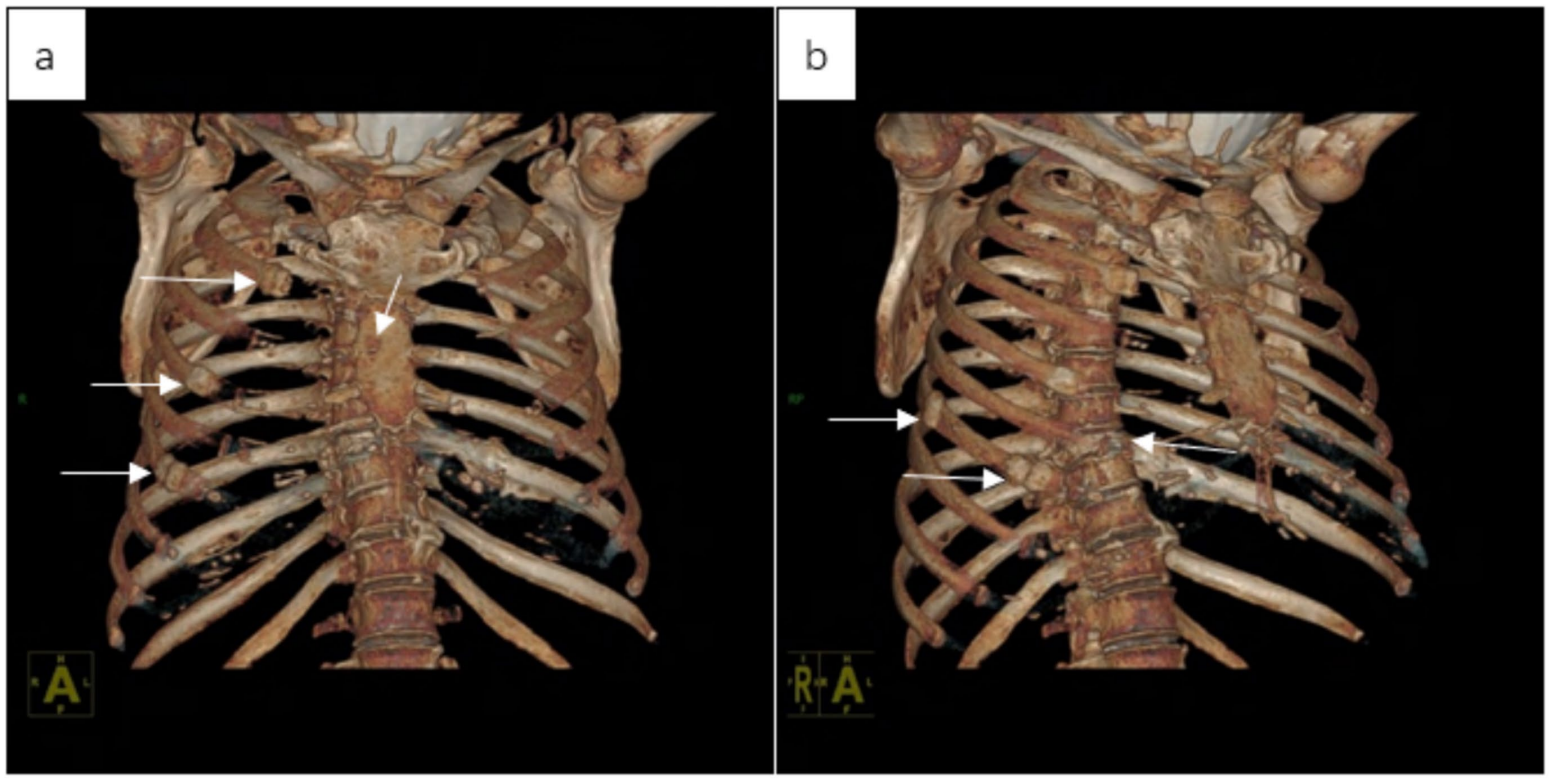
CT (bone window) of the chest and thoracic spine demonstrates postoperative absence of the left ninth rib consistent with prior resection. (a) Coronal image shows multifocal osteolytic lesions of the sternum and multiple bilateral ribs with pathologic fractures and callus formation. (b) Sagittal image shows vertebral osteolysis with compression fractures.
